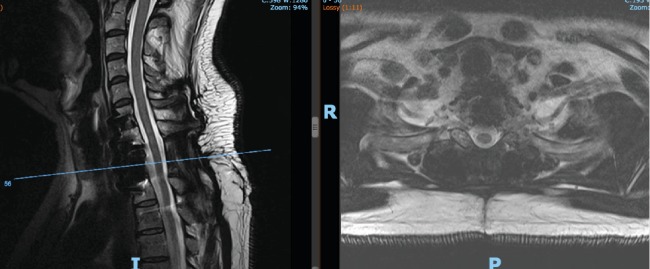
T2 magnetic resonance imaging at two-year follow-up appointment showing sagittal and axial views.
